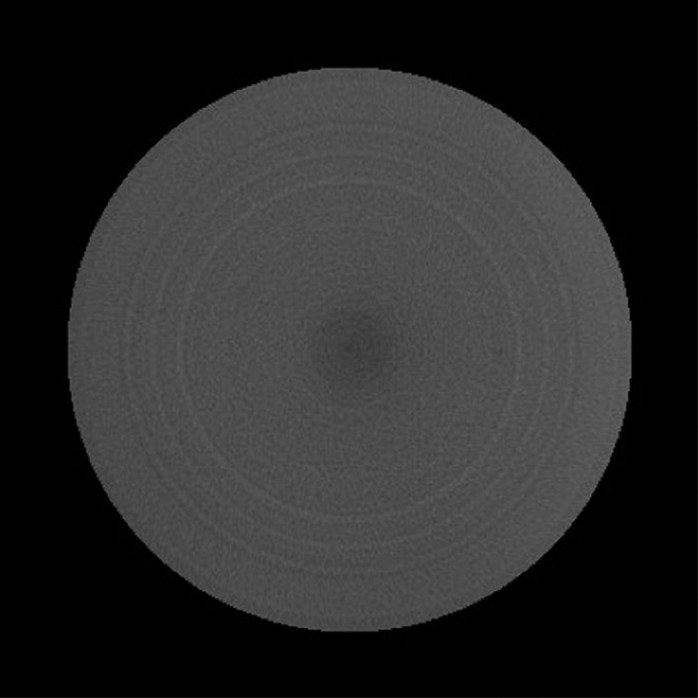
Trans‐axial cone beam computed tomography images of pelvis protocol with reversed half‐fan bowtie filter setup.
